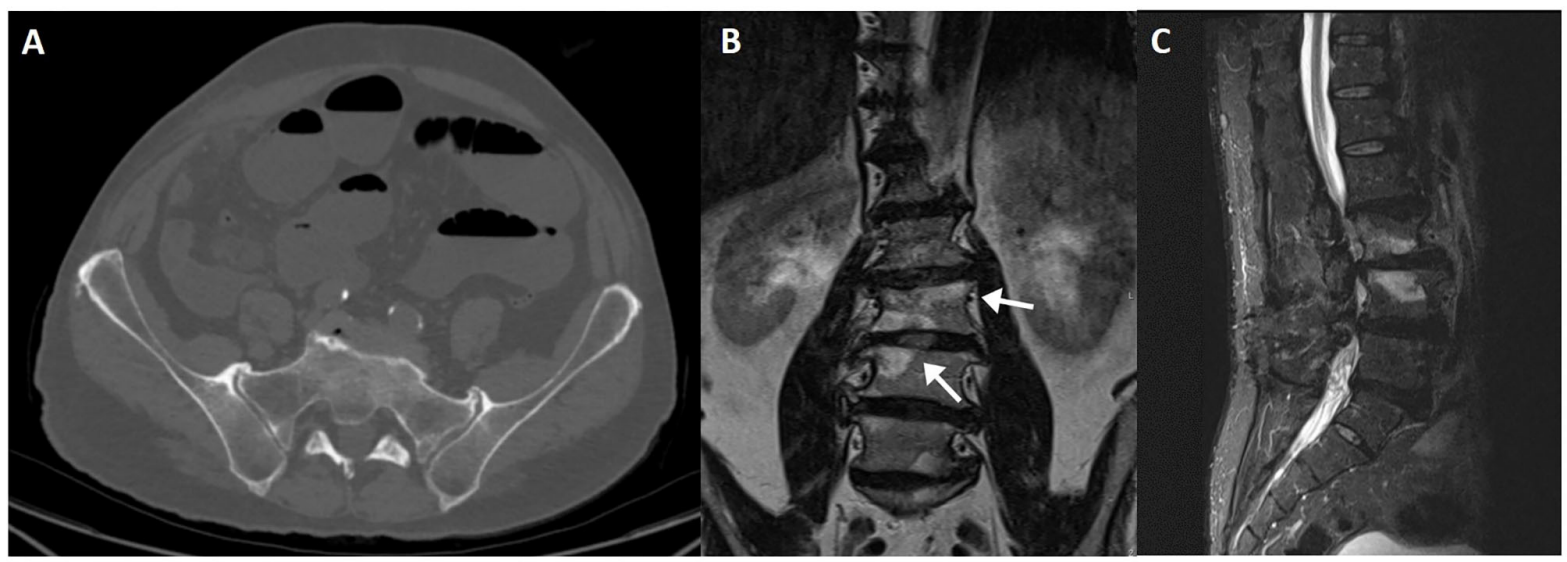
CT axial slice showing degenerative changes of the sacroiliac joint (A), with marginal osteophytes and bone sclerosis. Modic endplate changes at the weight bearing surfaces of the distal lumbar spine (arrows), seen on coronal T1 (B) and sagittal fluid-sensitive (C) sequences. Bone marrow signal changes are high on T1WI and fat-saturated T2WI, compatible with Modic type 2.
